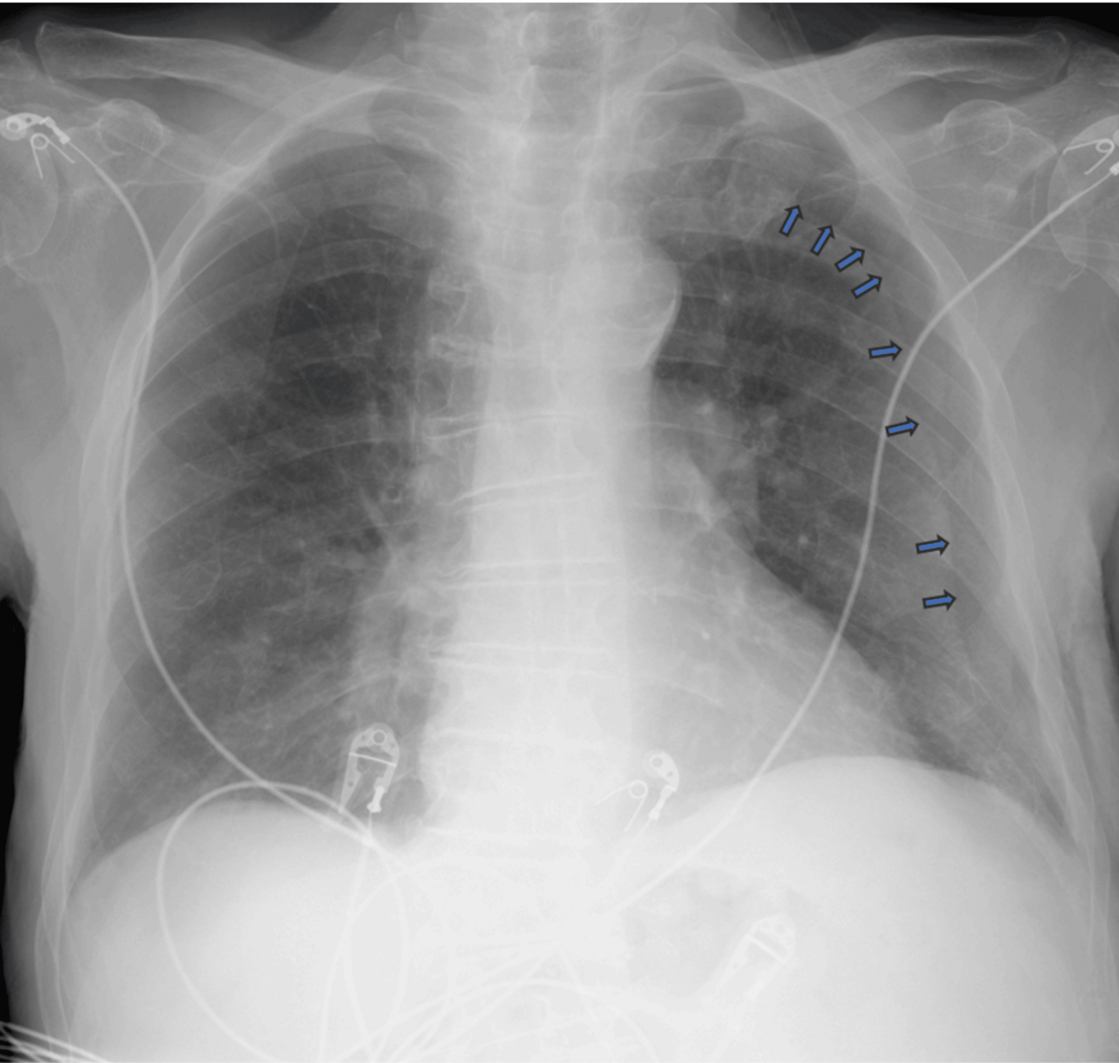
Anteroposterior chest X-ray showing a left skinfold, mimicking a left-sided pneumothorax. Blue arrows highlight the skinfold lining course starting as curved superiorly and becoming linear.
